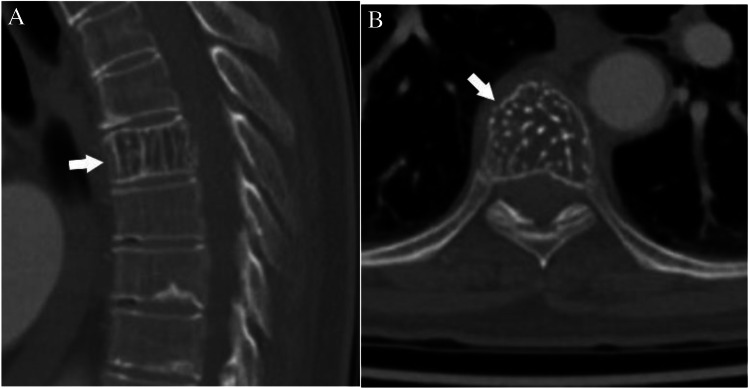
Case 10. Hemangioma. 73-year-old female with chest pain. Chest CT reveals an incidental T7 lesion. The lesion (arrows) has vertical striations on the A sagittal CT image and results in a “polka dot” appearance on the B axial CT image
